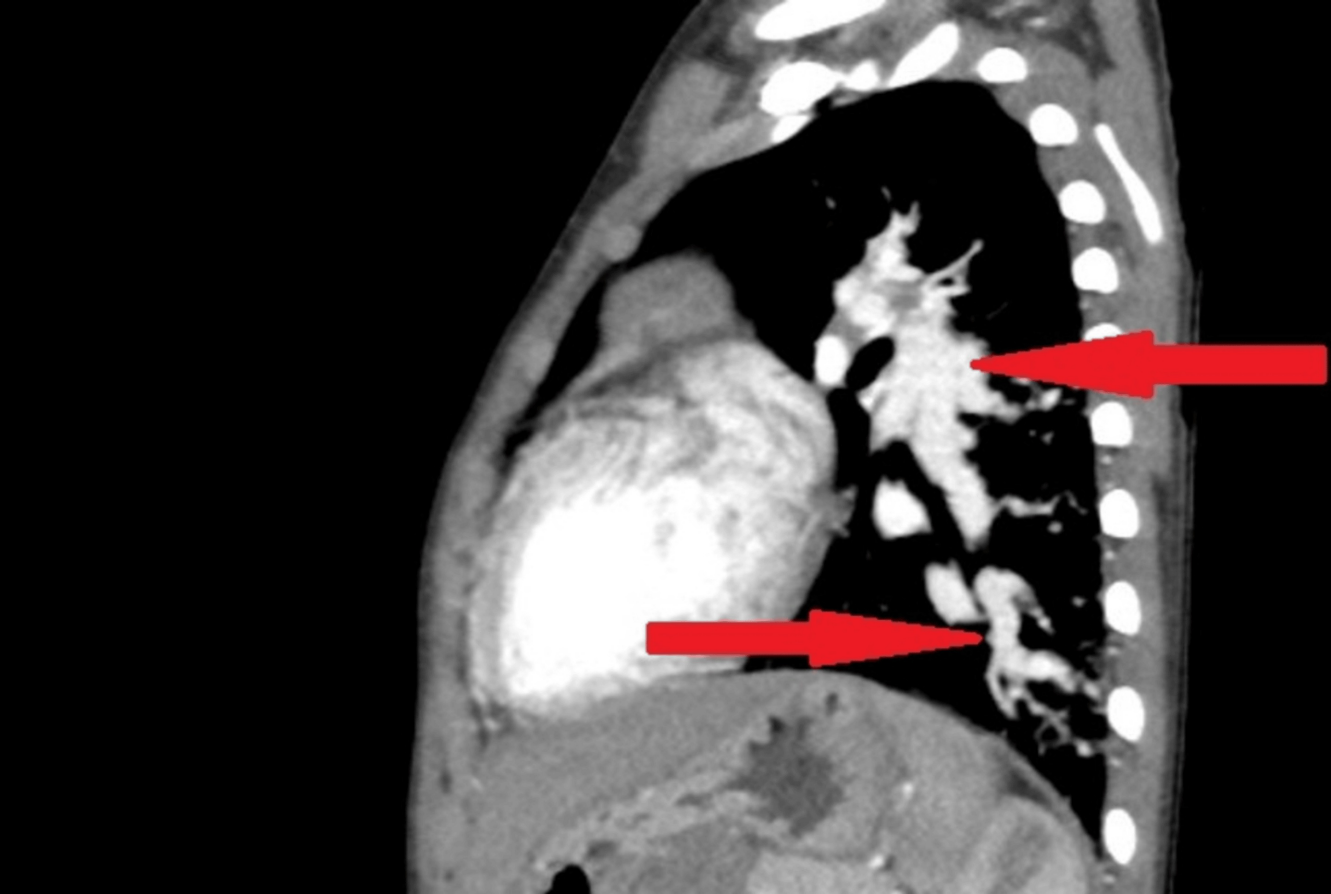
CT angiography of the thorax revealing multiple pulmonary arteriovenous malformations (red arrow)
